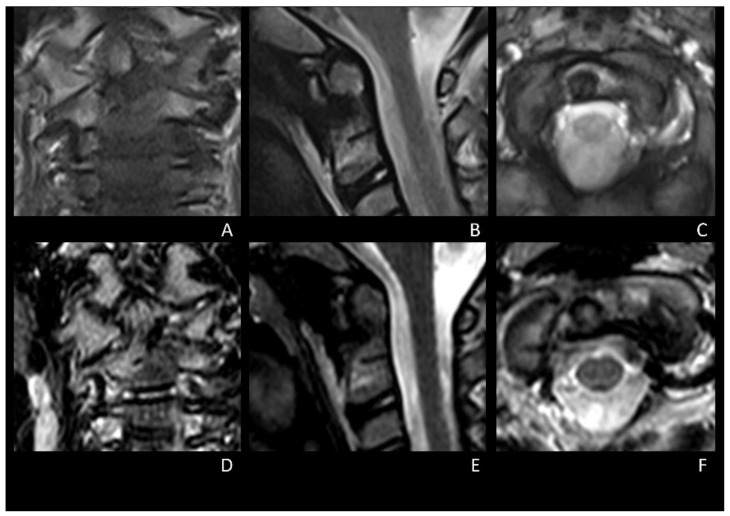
4-months (A–C) and 6-months (D–F) post-operative cervical MRI showing progressive and regular bone healing, without indirect signs of cervical instability and no relapse of OO.
